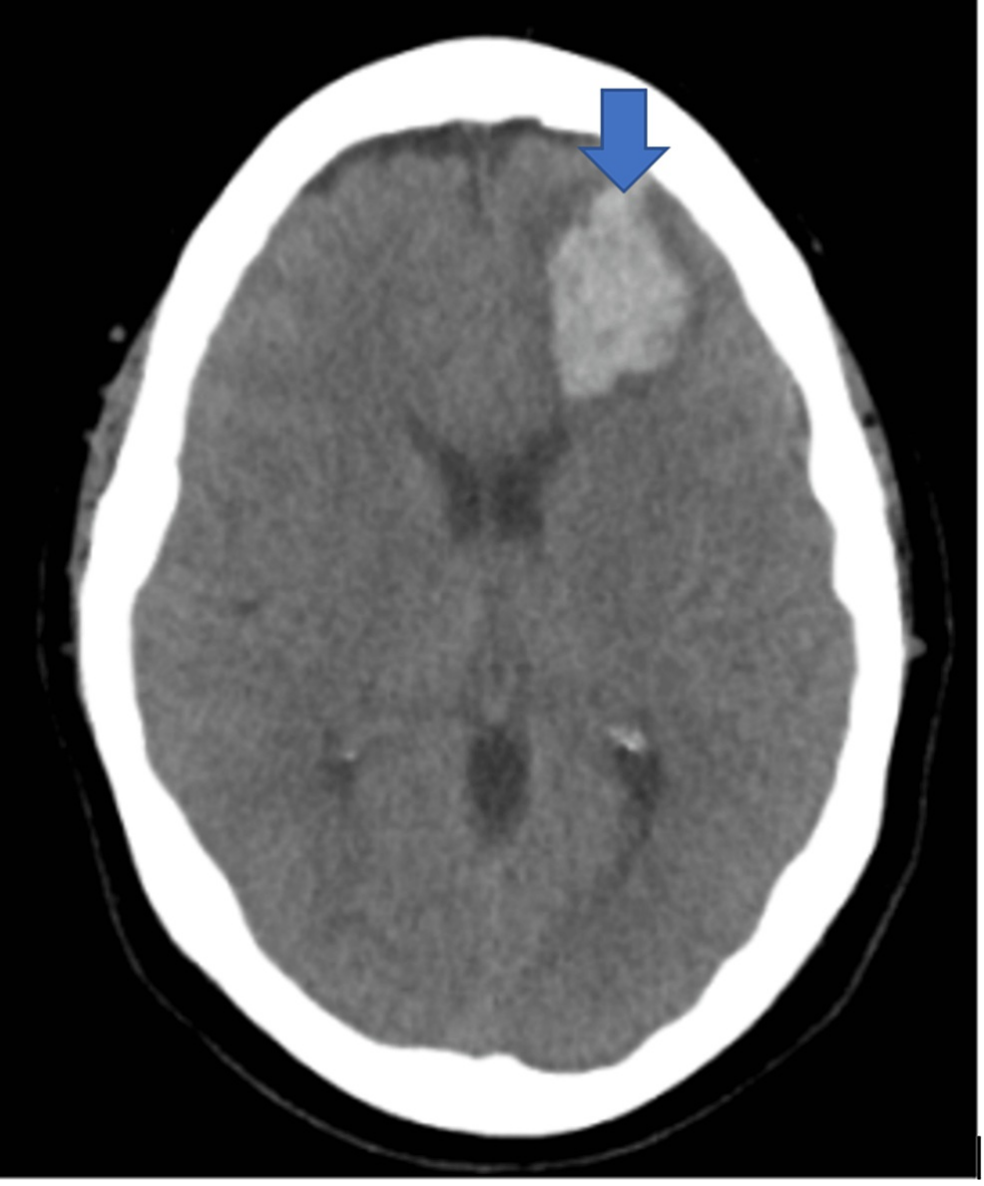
CT Head without contrast in Case 2 demonstrating new left frontal cortical intraparenchymal hemorrhage.
